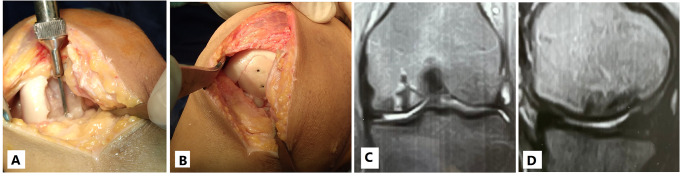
35 years old male right knee. A: Intraoperative image showing osteochondral defect of the medial femoral condyle measuring 27 mm * 23 mm. B: Shell osteochondral allograft fixed by two headless compression screws. C and D: 7 months postoperative MRI showing 75–100% cartilage fill with good contour
